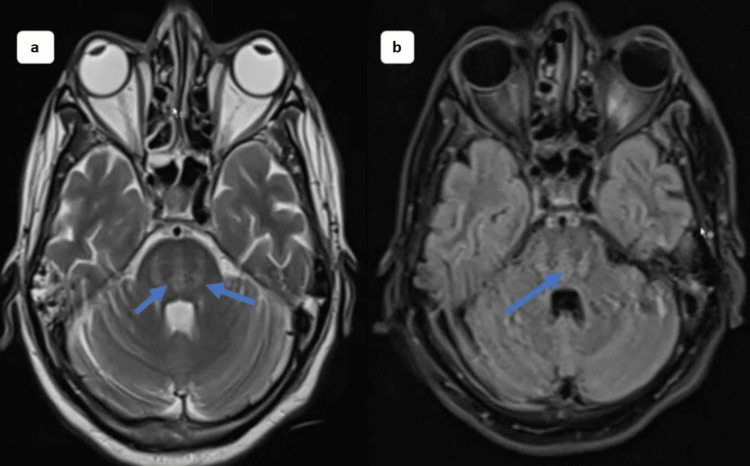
MRI brain demonstrating hyperintensity in the pons extending both sides of midline, suggesting demyelination (a, b). MRI: magnetic resonance imaging.
